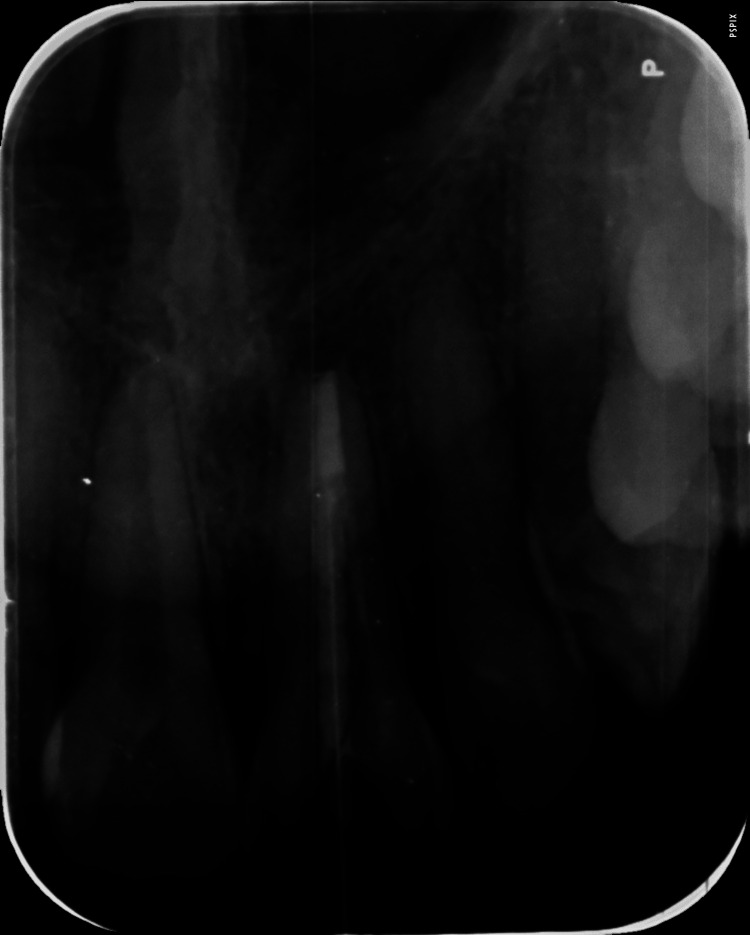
Radiographic evaluation of post fit with 21
